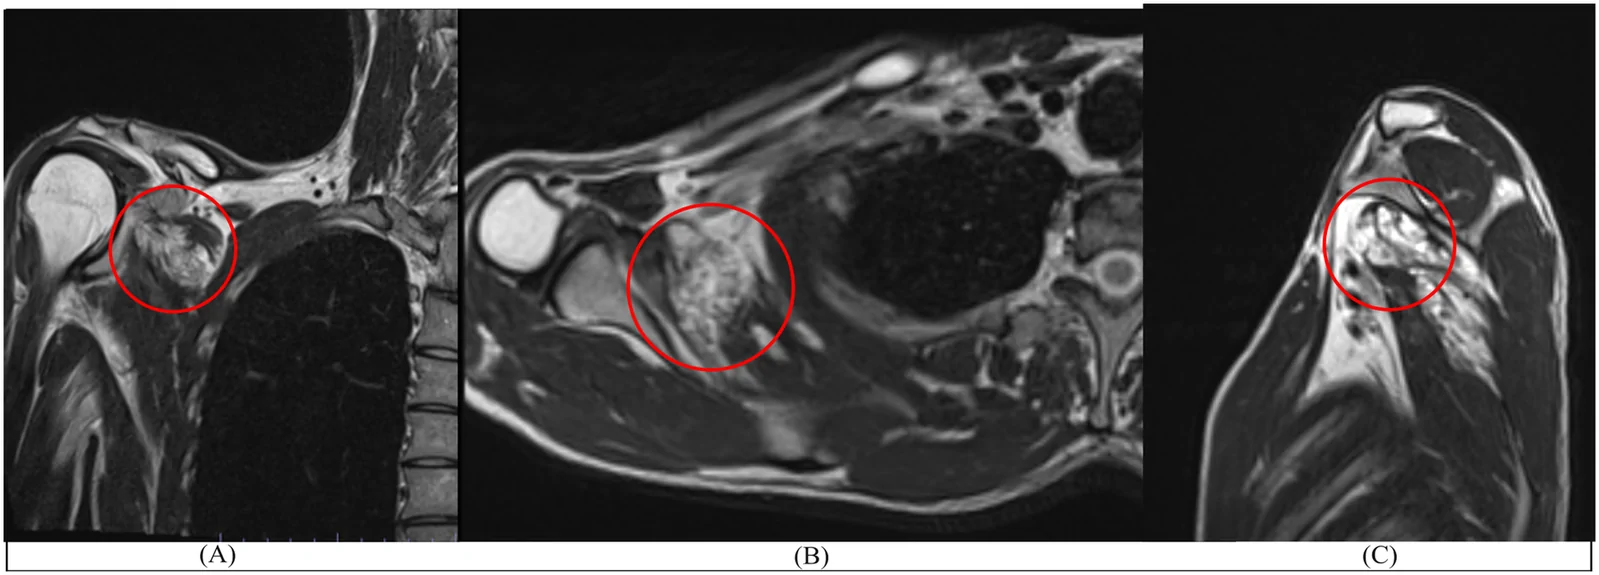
Contrasted MRI (after 2nd sclerotherapy) size of the lesion was marginally smaller 2.6 cm × 6.0 cm × 7.7 cm with clear fat plane seen between the venous malformation and the brachial plexus cords (red circle).
